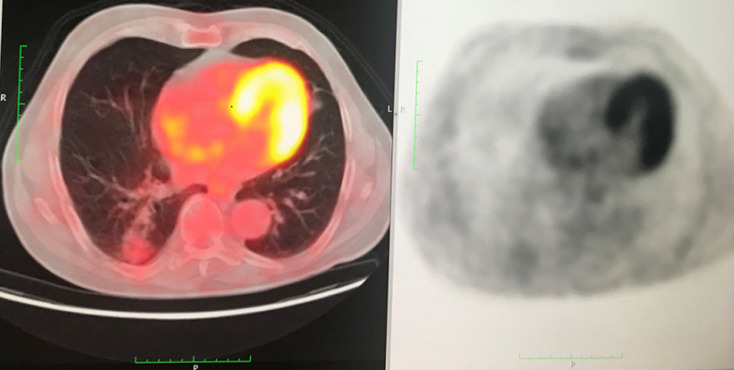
low grade FDG uptake in PET-CT (SUV: 1, 8)
